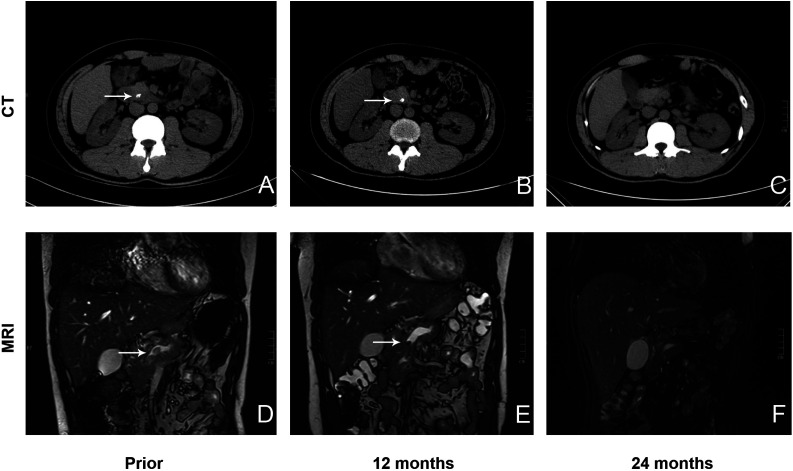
Dissolution of PDS with oral ECGG in a patient who had previously undergone ERCP and pancreatic sphincterotomy followed by balloon basket. (A) Cross-section CT image of the pancreas directly before treatment showing a relatively large stone in the head of the pancreas (arrows). (B) After oral ECGG treatment for 12 months, CT cross-section image showed a reduction in the size of PDS (arrows). (C) After oral ECGG for 24 months, CT cross-section image showed complete dissolution of PDS. (D) Magnetic resonance imaging coronal images of the pancreas directly before treatment showed a 0.9 cm diameter stone and many small stones in the pancreas head. (E) After oral ECGG treatment for 12 months, MRI coronal images showed a reduction in size and number of stones (arrows). (F) After oral ECGG treatment for 24 months, MRI coronal images showed complete dissolution of PDS. PDS, pancreatic duct stones; ECGG, endothelium corneum gigeriae galli; CT, computed tomography; MRI, magnetic resonance imaging; ERCP, endoscopic retrograde cholangiopancreatography.
